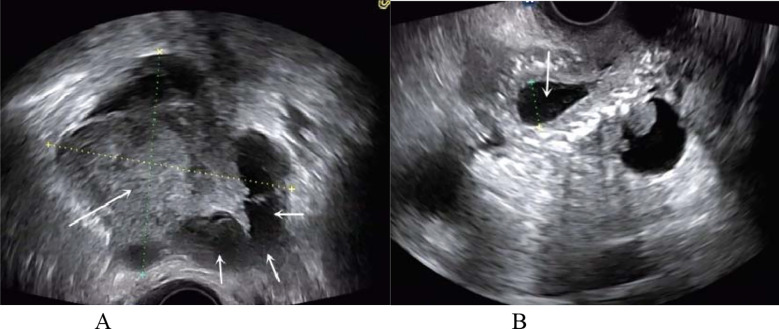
A patient with left type II PFTC (No. 14). (A): the transvaginal two-dimensional ultrasound showed the irregular and heterogeneous hypoechoic mass (long arrow) in the left adnexal region, with the dilated ureter (short arrow) adjacent to it; (B): the ultrasound showed the uterine fluid accumulation (arrow).
